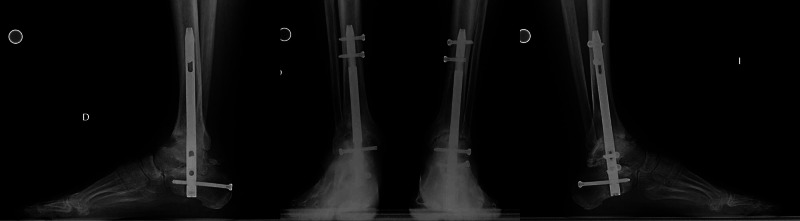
Postoperative weight bearing radiographs Three months after second surgery (right), weight bearing RX
